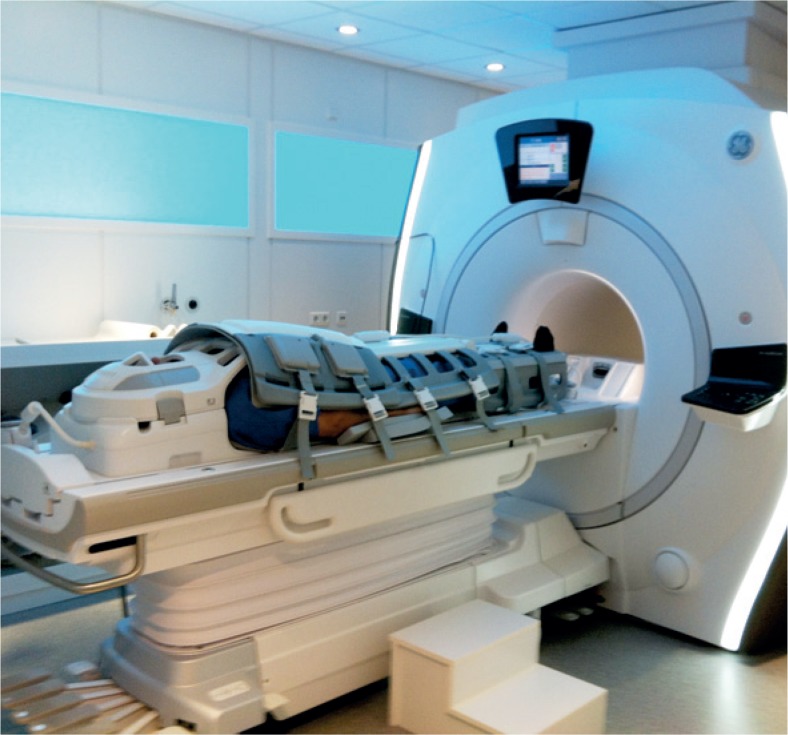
Whole-body MRI set-up of coils (head, chest, abdomen and extremity coils) in a wide bore magnet device.
